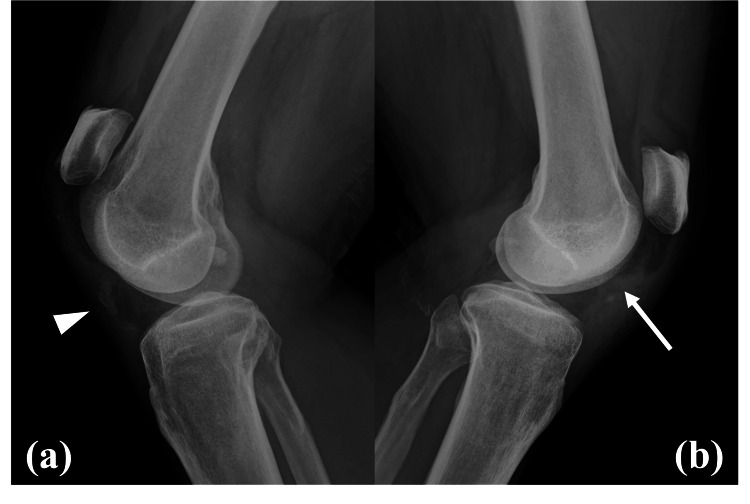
Preoperative lateral radiographs of the knees (a) right knee and (b) left knee. Both images demonstrate patella alta,  with increased density of the infrapatellar fat pad and loss of the sharp, linear margins of the patellar tendon. The arrowhead indicates marked calcification within the right patellar tendon, consistent with chronic degenerative changes, and the arrow indicates increased density of the infrapatellar fat pad.
